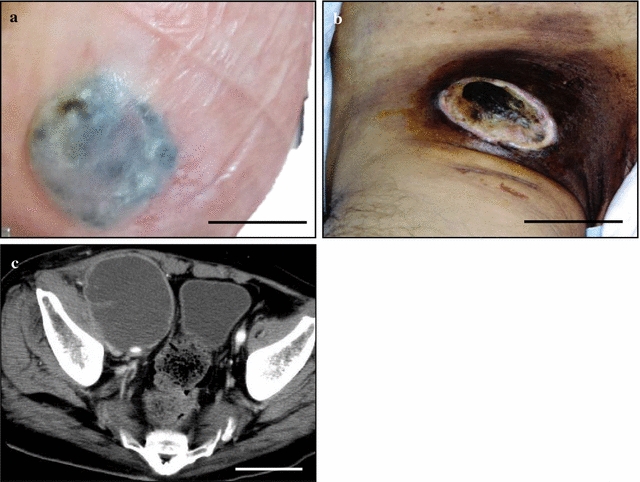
Macroscopic and imaging findings of this complete regression of cutaneous MM on the right sole associated with right inguinal lymph node metastasis. a, b The patient had a solitary bluish to *black patch* with surrounding depigmentation, accompanied by recent decrease in size, measuring up to 20 mm in the *right sole*. In fact, he had noticed it as a gradually growing, hemorrhagic and verrucous hypopigmented macule since approximately 2 years before. *Bar*  2 cm. b In parallel, he suffered from a rapidly growing and painful black-pigmented mass lesion with surface skin ulcer at the right inguen especially for recent 2 months, partly extending to the right scrotum and thigh. *Bar*  5 cm. c A pelvic CT scan revealed a relatively well-defined huge and low-density mass with peripheral enhancement, measuring approximately 10 × 8 cm. *Bar*  5 cm
